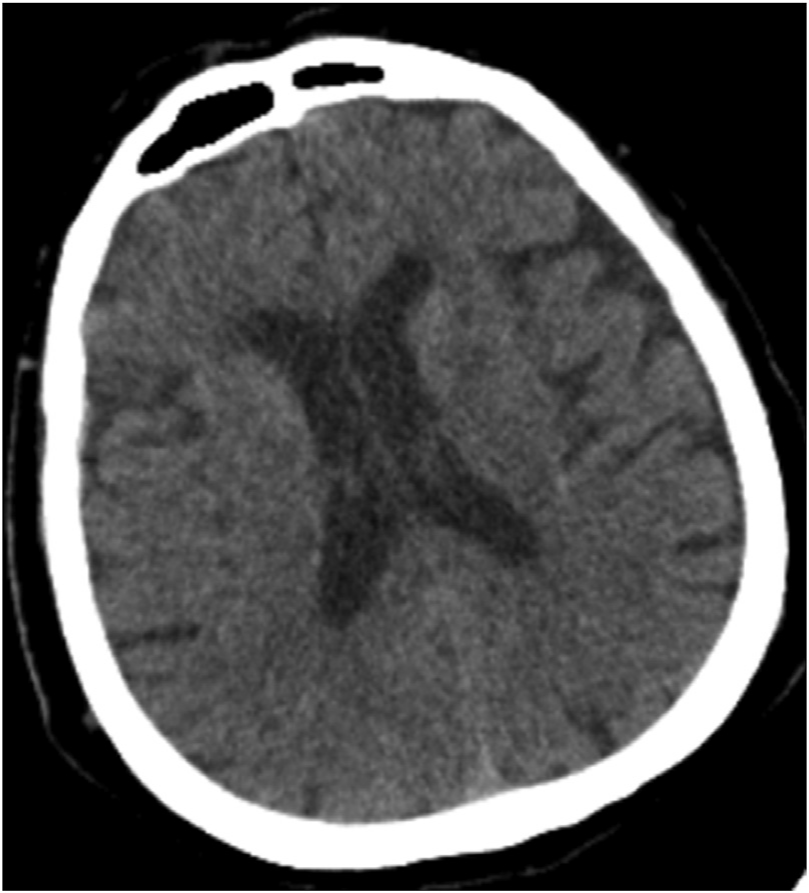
First admission day, brain CT scan showing no haematoma.
